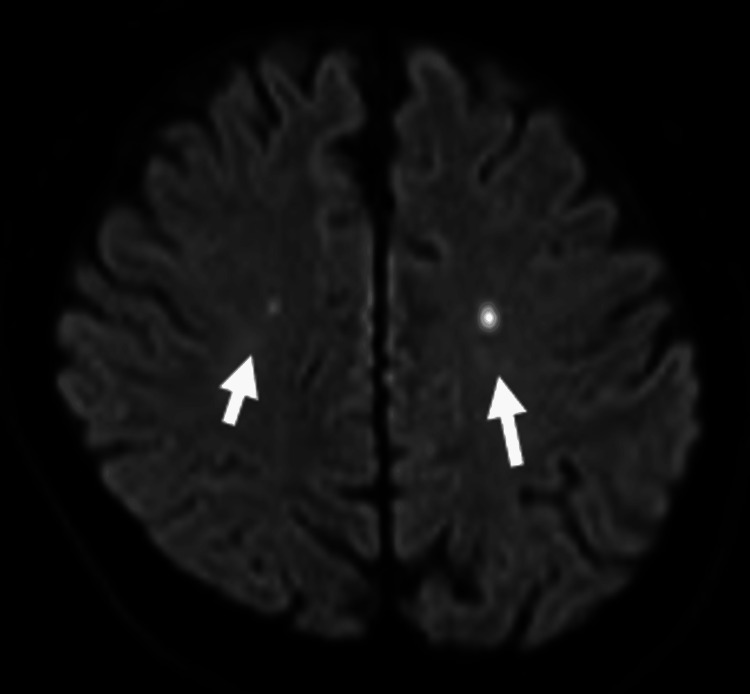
MRI of the head showing multiple acute cerebral microinfarctions (indicated by arrows). Diffusion-weighted magnetic resonance imaging of the patient’s head showing multiple acute cerebral microinfarctions caused by infective endocarditis.
